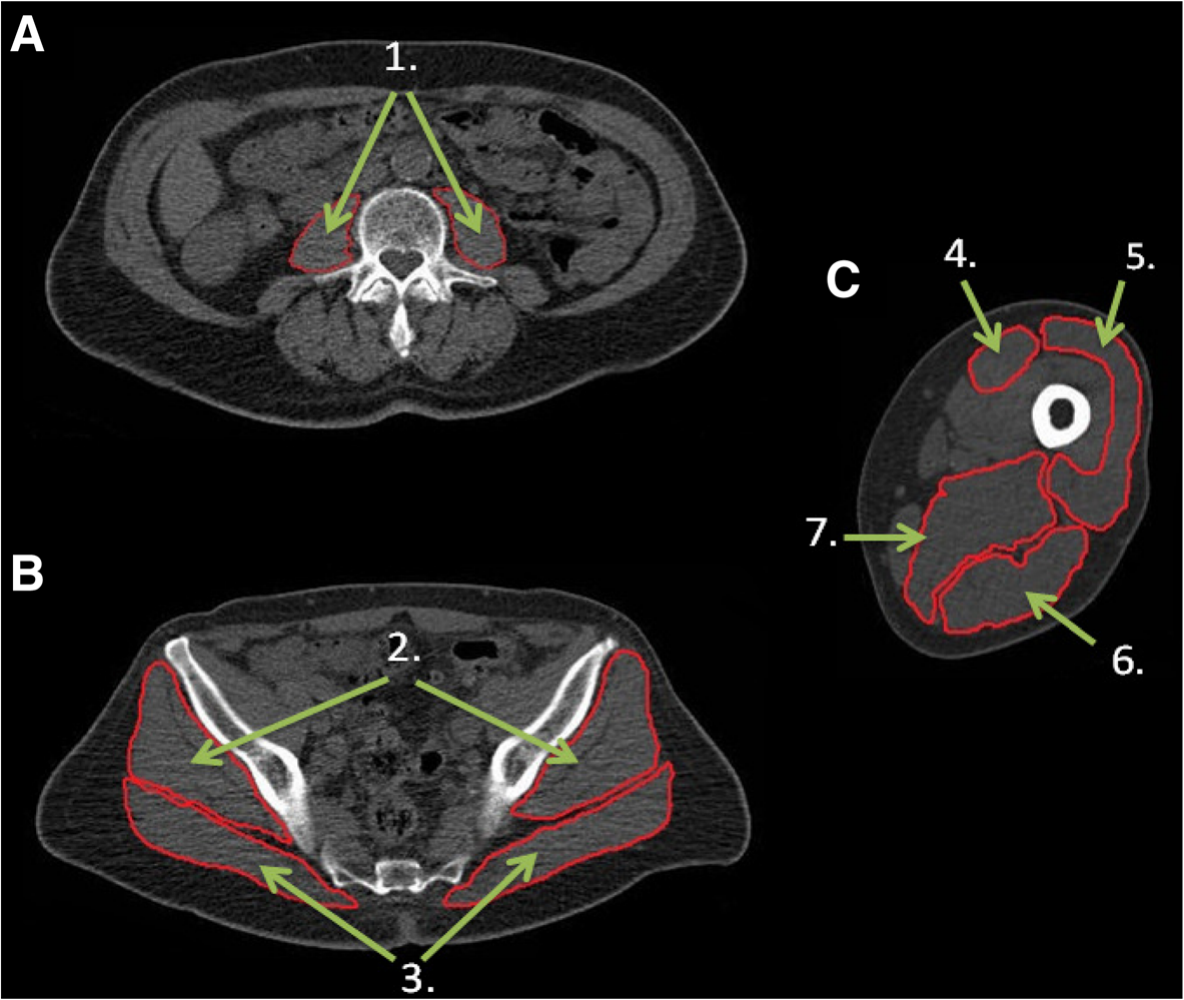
Representative example of computed tomography (CT) scans from an older adult showing A) abdominal scan; B) hip scan; C) thigh scan. 1. Psoas; 2. Gluteus Medius and Minimus; 3. Gluteus Maximus; 4. Rectus Femoris; 5. Vastus Lateralis; 6. Hamstrings compartment; 7. Adductor Magnus and Longus.
